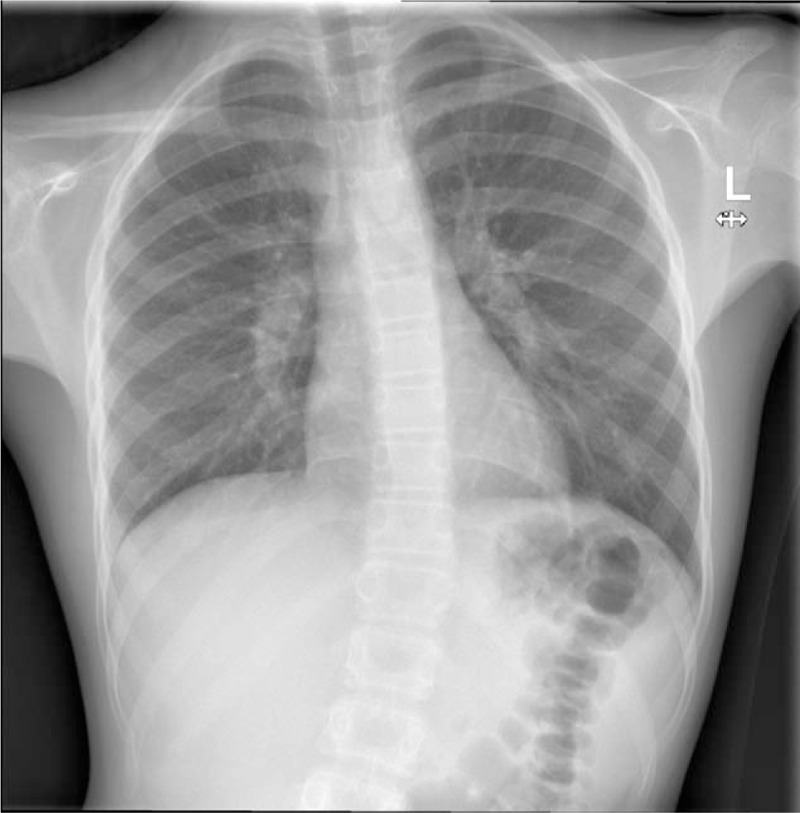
Normal chest radiograph on first presentation to hospital.
